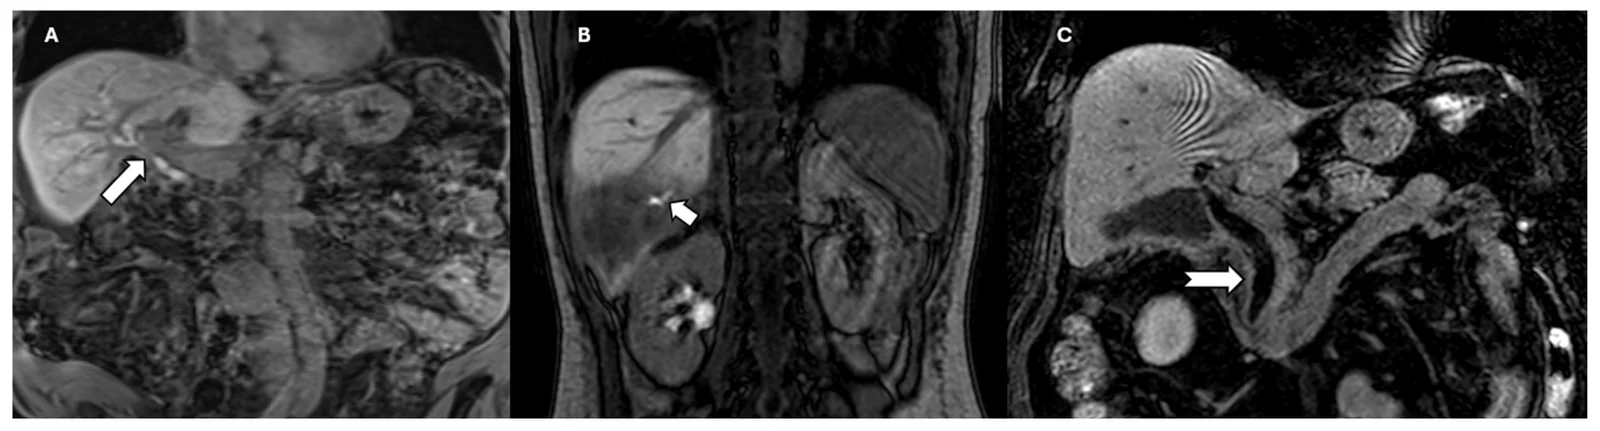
Representative clinical applications of functional hepatobiliary MRI. (A) Coronal hepatobiliary phase MRI image showing biliary obstruction at the hepatic hilum (long arrow), with distal contrast excretion. (B) Coronal hepatobiliary phase MRI image demonstrating a post-traumatic bile leak (short arrow), with hepatobiliary contrast extravasation into a perihepatic fluid collection. (C) Coronal hepatobiliary phase MRI image showing markedly impaired hepatobiliary contrast excretion associated with dilatation of the common bile duct (notched arrow), consistent with biliary outflow obstruction.
